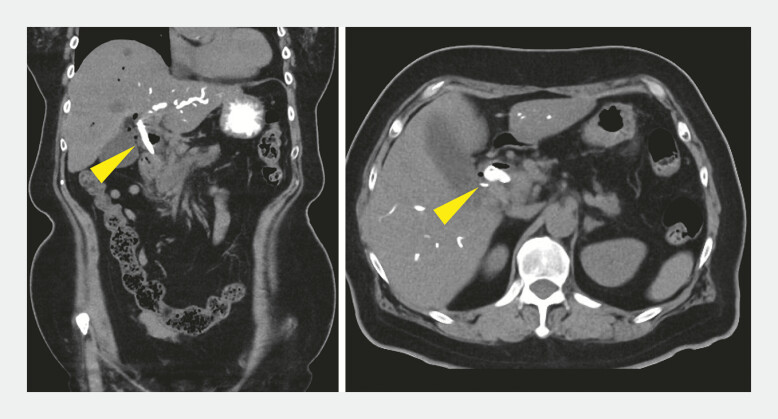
Post-procedure computed tomography scan demonstrating a properly positioned self-expandable metallic stent (yellow arrowhead) without any bile leakage.
